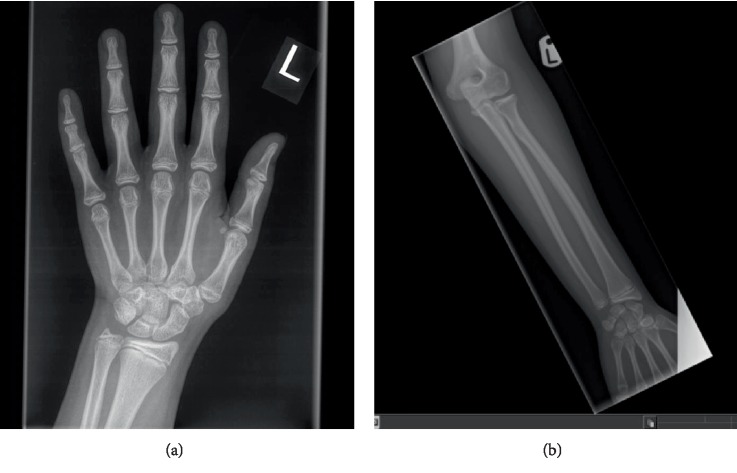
(a and b) Radiographs of A1's left hand and left forearm with changes described in the text.
